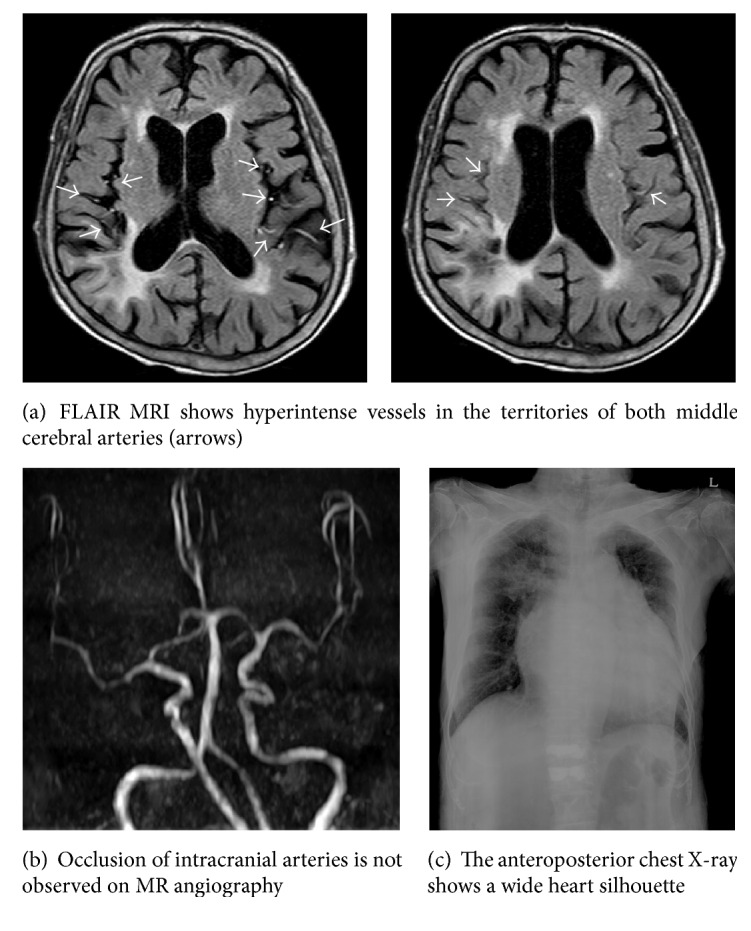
Magnetic resonance and chest X-ray images.
